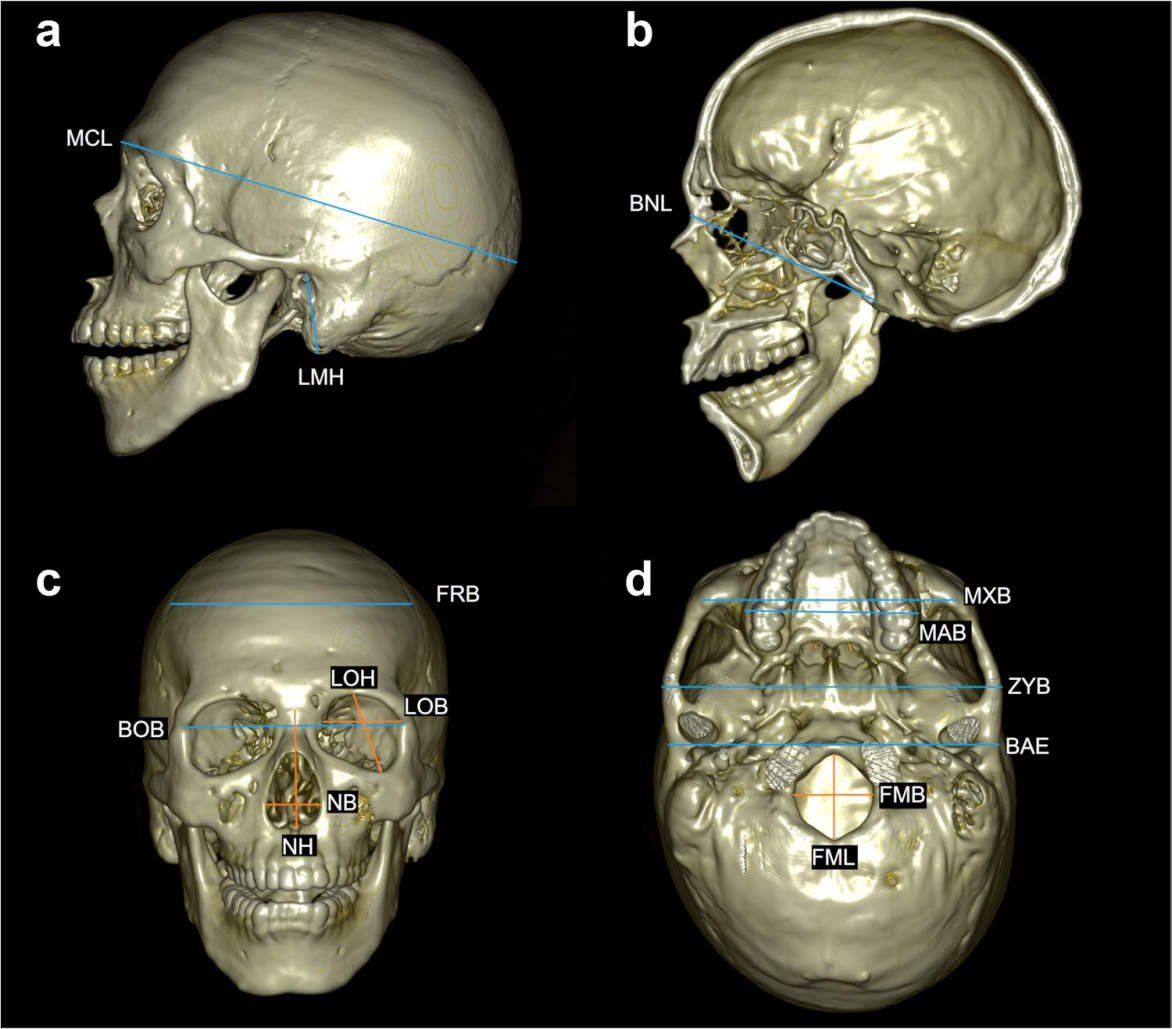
Three-dimensional computed tomography images showing cranial measurements (see Table 2 for definition): a maximum cranial length (MCL) and left mastoid height (LMH); b basion-nasion length (BNL); c frontal breadth (FRB), biorbital breadth (BOB), left orbit height (LOH), left orbit breadth (LOB), nasal height (NH), and nasal breadth (NB); d bimaxillary breadth (MXB), maxillo-alveolar breadth (MAB), bizygomatic breadth (ZYB), biauricular breadth (BAE), foramen magnum length (FML), and foramen magnum breadth (FMB). Right mastoid height (RMH), right orbit height (ROH), or right orbit breadth (ROB) is not shown because they are just left symmetrical
